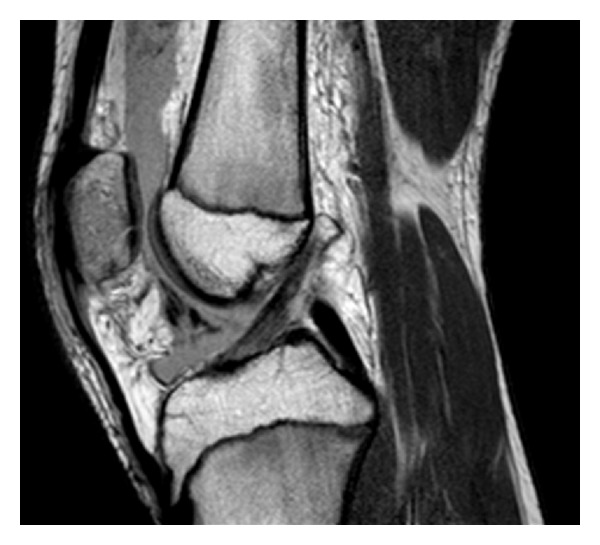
Sagittal T1 weighted magnetic resonance image taken one week after presentation.
